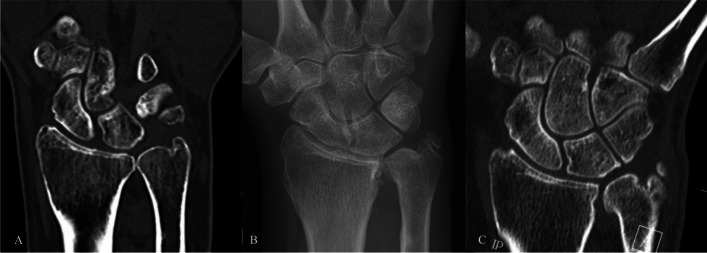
Preoperative CT in case 4 with an obliterated joint space in the DRUJ (A). Postoperative CR with an increased distance in the joint (B), which is confirmed with CT 2.5 years postoperatively (C)
